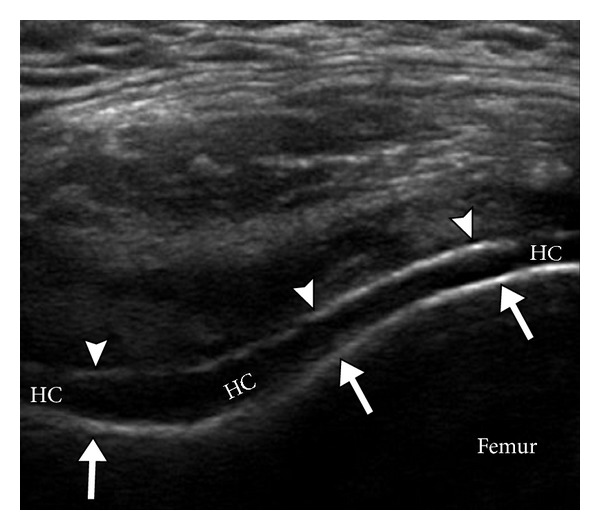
Ultrasound double contour sign. Transverse ultrasound image of the suprapatellar knee joint demonstrates two parallel hyperechoic contours on either side of the hypoechoic hyaline cartilage (HC). The deep echogenic contour (long arrows) represents the femoral cortex, while the superficial echogenic contour (arrowheads) represents uric acid crystals accumulating on the surface of the hypoechoic hyaline cartilage (HC).
